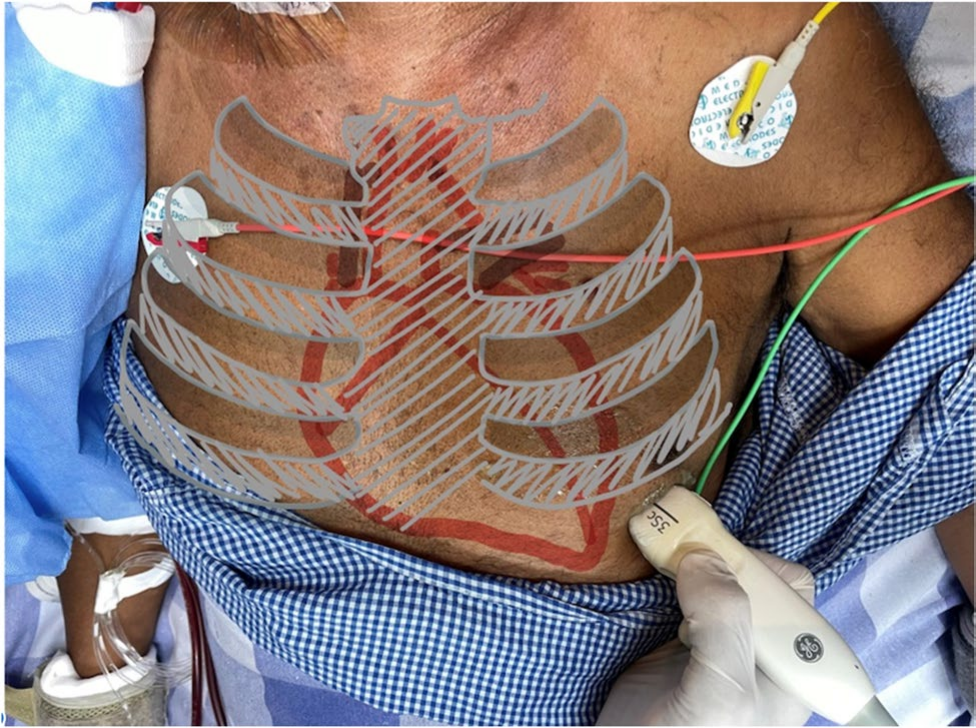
Probe placement for Apical 4 chamber view. Probe placed at the apex to obtain the 4- chamber view for TAPSE measurement
